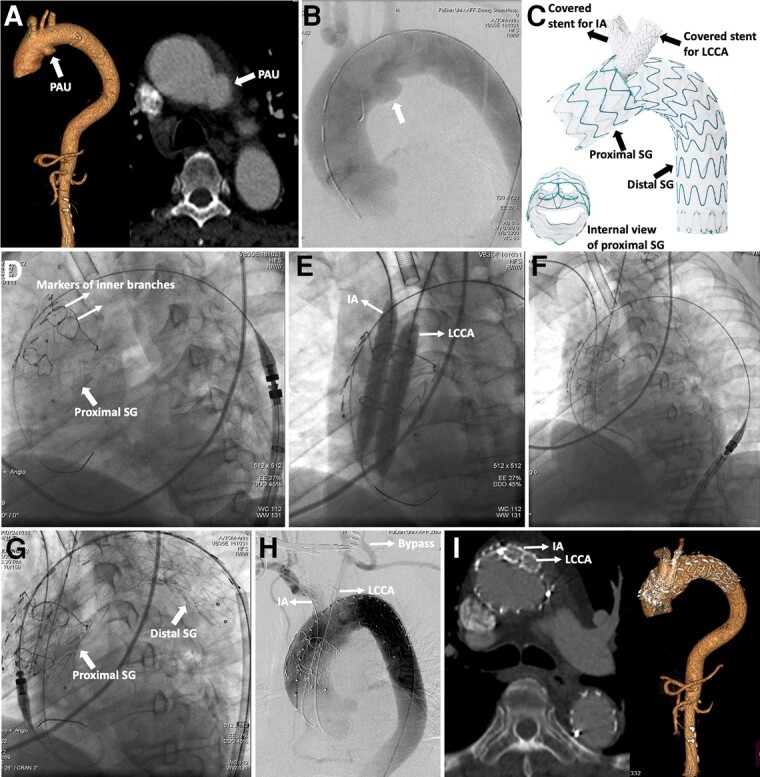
(*A*) Computed tomography angiography confirmed penetrating aortic ulcer in the aortic arch. (*B*) Digital subtraction angiography confirmed the diagnosis. (*C*) Design of the novel double inner-branched device. (*D*) Deployment of the stent-graft in the ascending aorta. (*E*) Confirmation of two inner branches with balloons. (*F*) Deployment of bridged covered stents in the innominate artery and left common carotid artery. (*G*) Deployment of the distal stent-graft. (*H*) Final angiography showed procedural success. (*I*) Computed tomography angiography was followed up for 6 months. PAU, penetrating aortic ulcer; IA, innominate artery; LCCA, left common carotid artery; SG, stent-graft.
